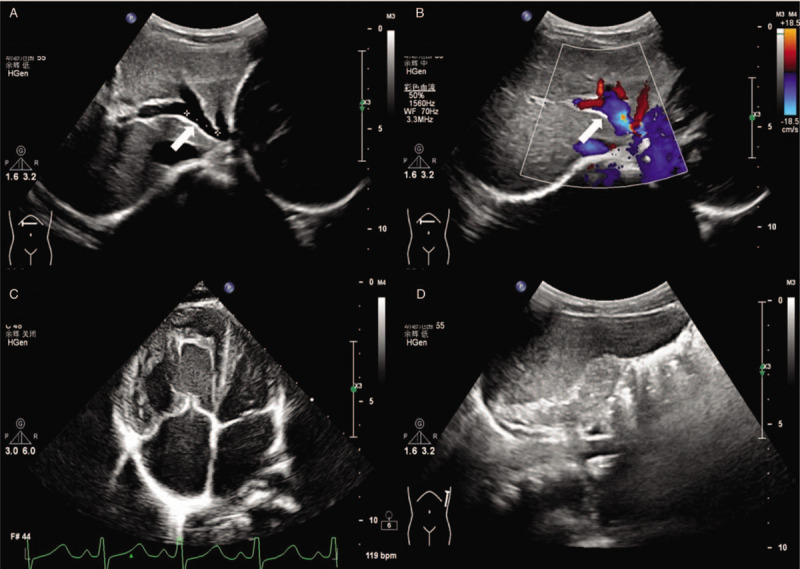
Abdominal ultrasound and echocardiogram. (A) the main branch of the portal vein and the patent ductus venosus can be seen, (B) the blood flow of patent ductus venosus can be seen, white arrow indicating ductus venosus, (C) echocardiography shows the enlargement of right ventricle and right atrium, (D) the enlargement of spleen can be seen.
